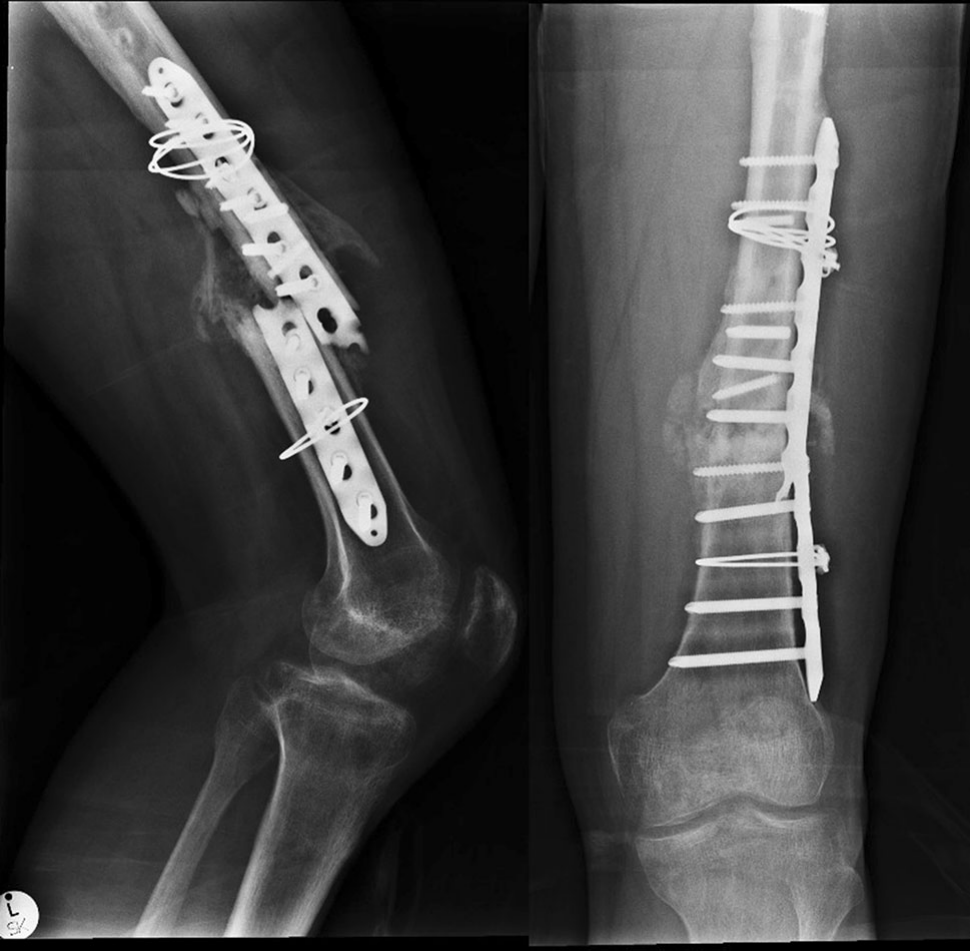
Anteroposterior and lateral radiographs of the femur demonstrating angulation, nonunion and failed locking plate at the fracture site
